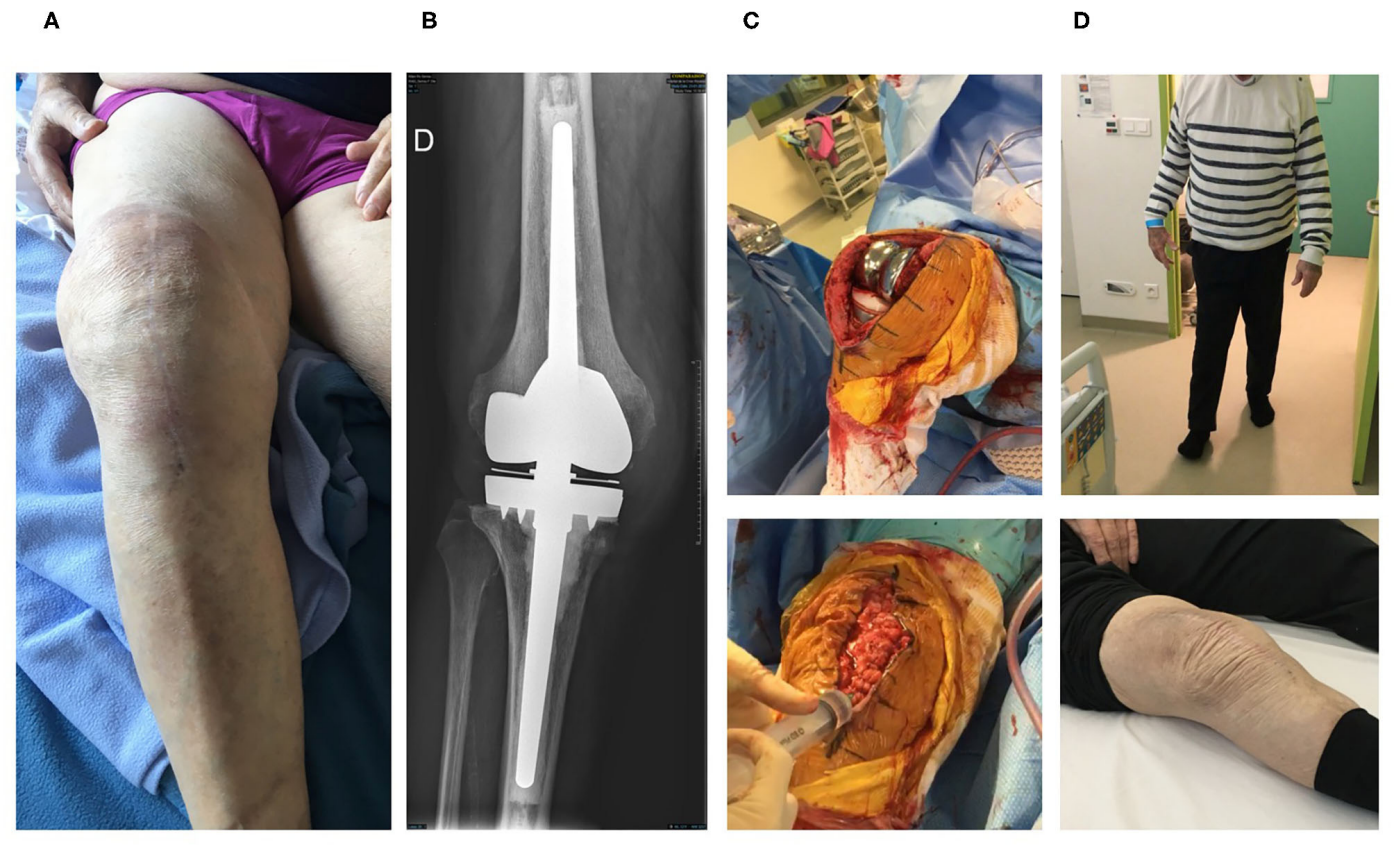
The second patient is an 84-year-old man with past history of dyslipidemia and right prosthetic-knee arthroplasty in 2006. A two-stage exchange was performed in 2007 for a *S. epidermidis* PKI. As the patient kept a painful knee, a one-stage exchange was performed in 2016, with implantation of a hinged cemented knee prosthesis with long stem. Cultures remained sterile. In 2019, the patient developed clinical signs of acute septic arthritis with fever. A DAIR without polyethylene exchange was performed and methicillin-susceptible *S. aureus* grew in perioperative samples and blood cultures. Endocarditis was excluded. The patient was treated using intravenous cefazolin and rifampin orally. Cefazolin was switched to ofloxacin 3 weeks after the DAIR and was combined with rifampin. Under this treatment, new signs of septic arthritis occurred 6 weeks after the DAIR, with local erythema, pain, and large joint effusion (A). A joint puncture was performed, but no pathogen was isolated in cultures, and the failure was attributed to *S. aureus*. X-ray showed no loosening of the prosthesis (B). Open DAIR was performed showing significant local inflammation and pus into the joint. The personalized cocktail of phages was administered after joint closure (C). The patient improved quickly, doxycycline was then prescribed as suppressive therapy, and the outcome was favorable at 7 months with no signs of infection, a negative C-reactive protein and pain-free walking [(D), Supplementary Video 2].
